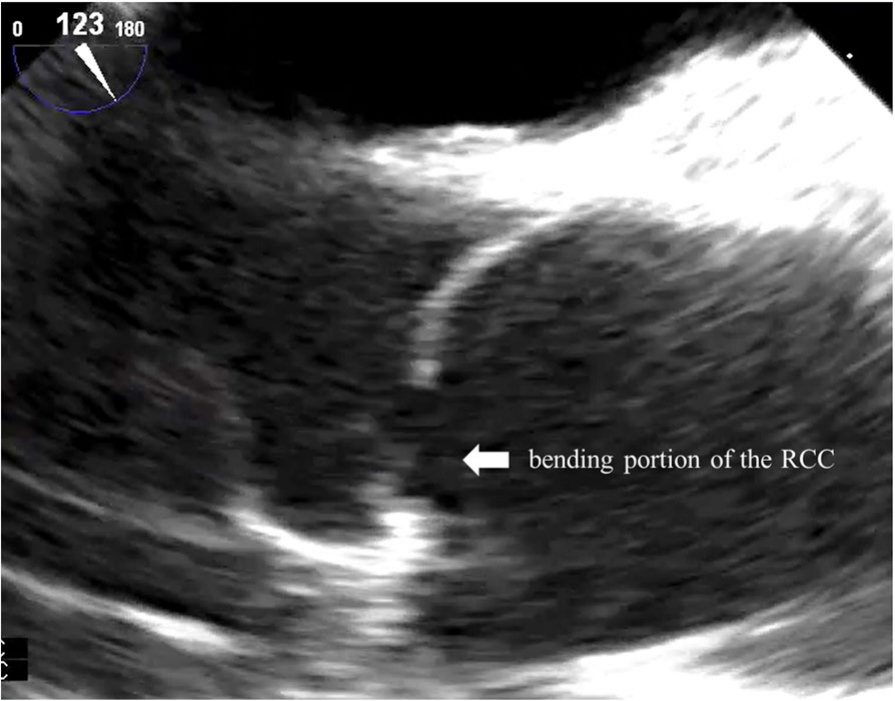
Preoperative transesophageal echocardiography images. Preoperative transesophageal echocardiography revealing that the main cause of AR was suspected to be RCC prolapse (type II)
